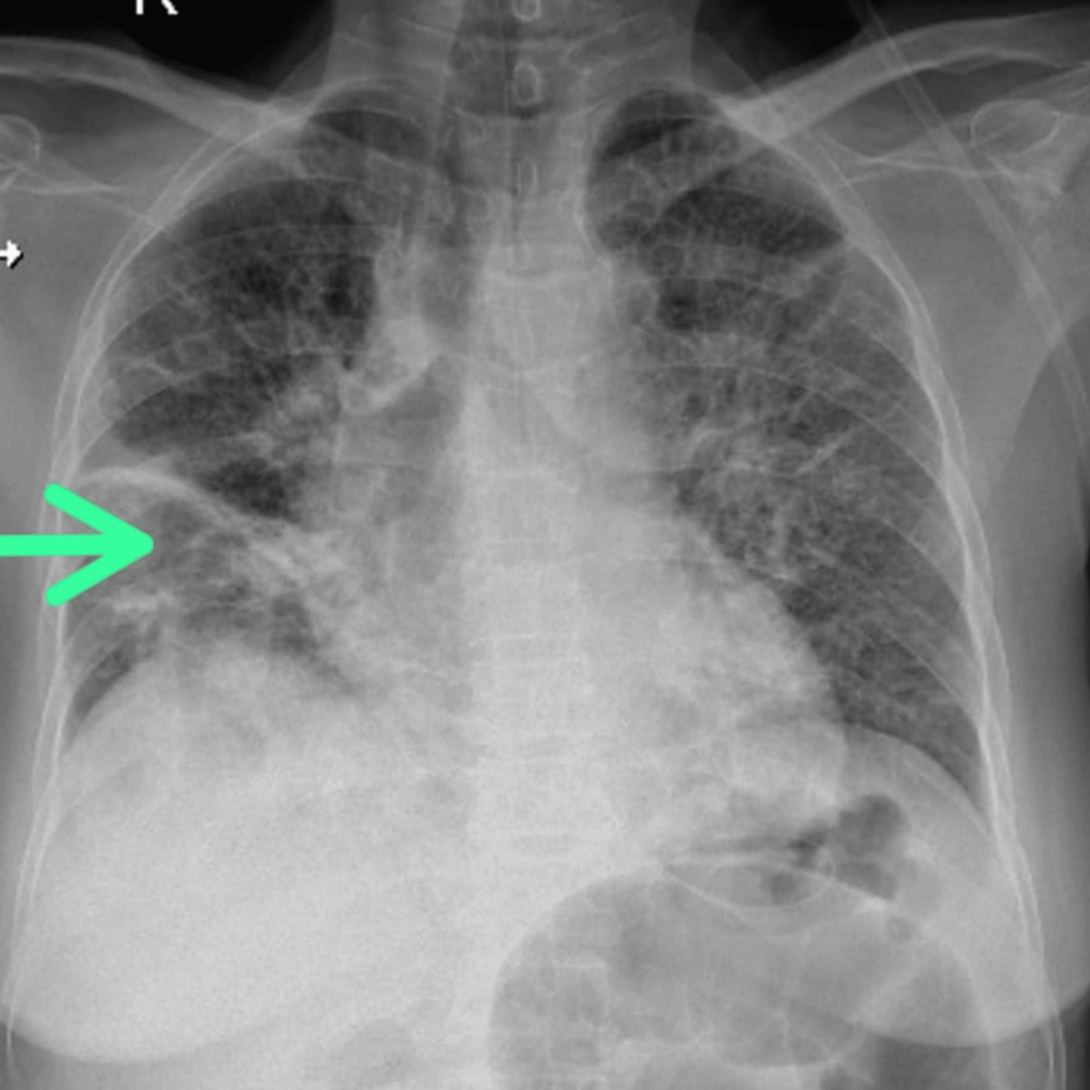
Plain Radiograph of the Chest
